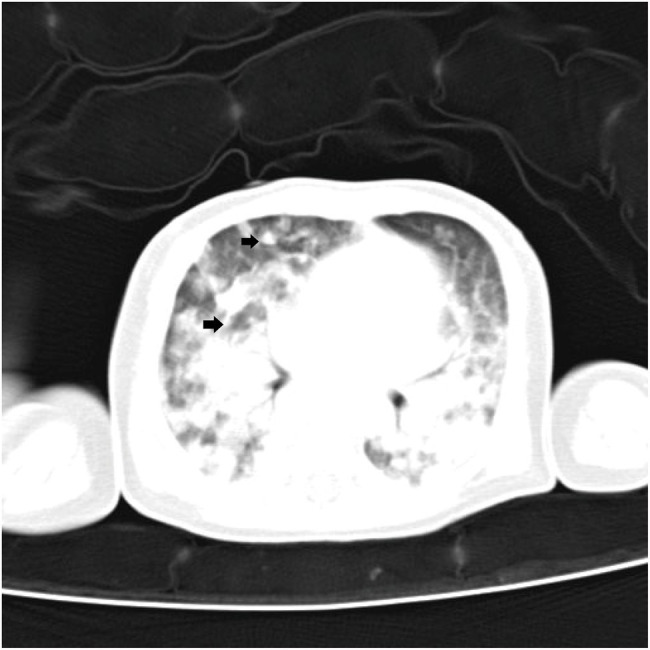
Chest computed tomography image of patient 1 showing many miliary nodules (arrowheads) can be seen in both lungs.
